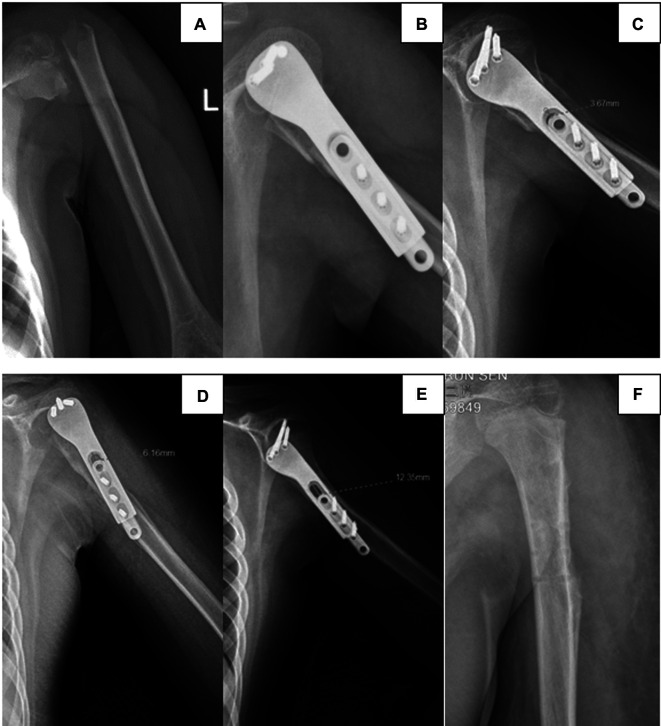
Radiographs of a proximal fracture of the left humerus in a 9-year-old boy by a traffic accident. (A) Preoperative lateral radiography shows proximal humeral fractures, Neer–Horwitz grade IV. (B) Intraoperatively, the internal fixation device is found to be in good position. (C) Lateral radiography at 6 weeks after surgery. (D) Lateral radiography at 3 months after surgery. (E) Lateral radiography at 6 months after surgery. (F) Postoperatively, slide plate removal.
